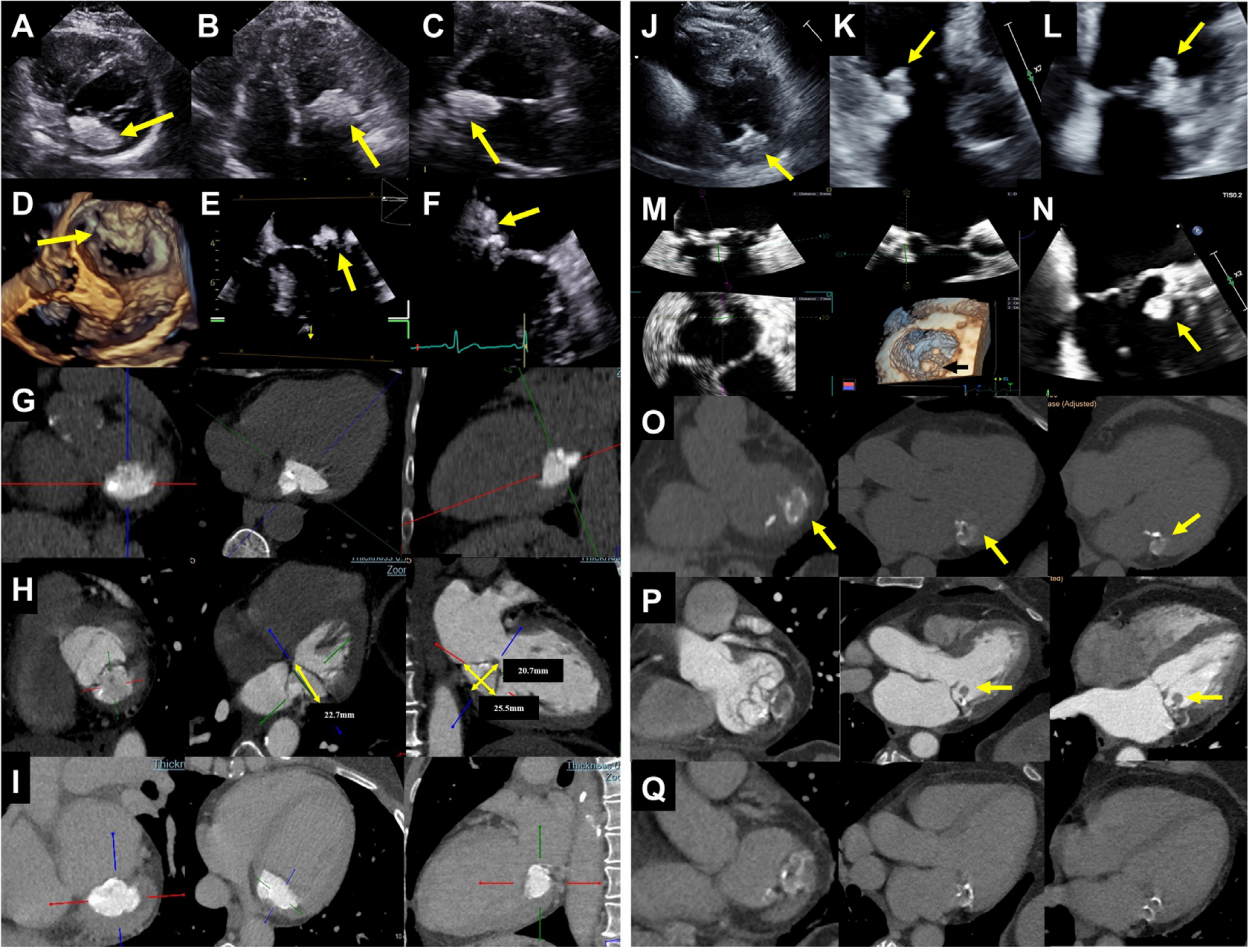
Multimodality Images Case 1 is presented in A to I in which short-axis, 4-chamber, and 2-chamber views are shown, respectively, with (A to C) transthoracic echocardiography (TTE), (D-F) transesophageal echocardiography (TEE), (G) multiplanar reconstrication (MPR) noncontrast cardiac computed tomography angiography (CTA), and (H) arterial and (I) venous phases. TTE short-axis view at the mitral valve annulus (MA) level shows an inferior-posterior calcified mass (A, yellow arrow). The mass is extended towards the left atrium as shown in the 4-chamber view (B, yellow arrow) and 2-chamber view (C, yellow arrow). The surgical view on 3-dimensional TEE (D, yellow arrow) and 2-dimensional 4-chamber (E, yellow arrow) and 2-chamber view (F, yellow arrow) emphasize the irregularity of the mass while protruding to the left atrium. Multiplanar cardiac CTA images with noncontrast, arterial and venous phase, are presented in G, H, and I, respectively. In noncontrast images, the caseous mitral valve annulus calcification (MAC) is hyperattenuated with nonhomogenous calcification, especially seen in the edges of the mass. Note the relatively hypodense core. The caseous MAC protrude extends both towards left atrial and ventricle and was measured in arterial phase 22.7 × 20.7 × 25.5 mm (H). Enhancement is not seen in both arterial and venous phase (H, I). Case 2 is presented in J to Q in which short-axis, 3-chamber, and 4-chamber views are shown, respectively, with TTE (J to L), TEE (M and N), noncontrast MPR cardiac CTA (O), and arterial (P) and venous phases (Q). The TTE short-axis view of the left ventricle shows a caseous MAC extension toward the left ventricle (J, yellow arrow). The attached mobile portion is shown in K and L (yellow arrow) at 3-chamber and 4-chamber views, respectively. The TEE multiplanar 3-dimensional TEE measuring the mobile component of the caseous MAC (9 × 7 × 10 mm) and a ventricle view of it is shown in M (black arrow). A 2-dimensional TEE, 4-chamber view of the caseous MAC including the mobile mass is shown in N. Establishment of caseous MAC diagnosis with cardiac CTA is shown in O to Q. (O) Shows the nonhomogenous calcified MA mass (yellow arrow) with noncontrast images. Note the relatively hypodense core. The mobile mass is better defined with the contrast images (P, yellow arrow). No mass enhancement is observed in both arteria (P) and venous (Q) phases.
